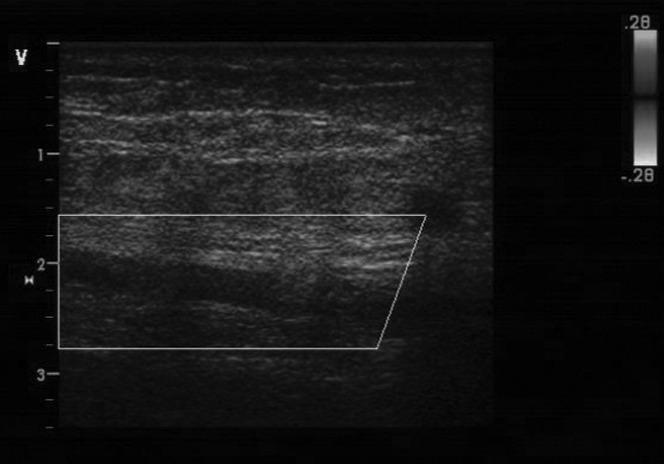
Color doppler ultrasound reveals that the brachial vein static wall is smooth and the thrombus has disappeared. Same patient as shown in Fig 1.
